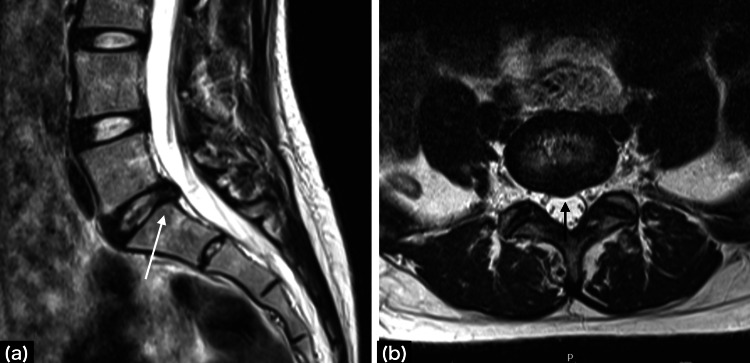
Magnetic resonance imaging T2-weighted images (a) Sagittal view; a posteriorly protruding disc at high L5/S1 is observed. The white arrows indicate mild HIZs, (b) Axial view of L5/S1; a posterior midline protruding herniated disc is observed. The black arrows indicate mild HIZs. HIZs: high-intensity zones
